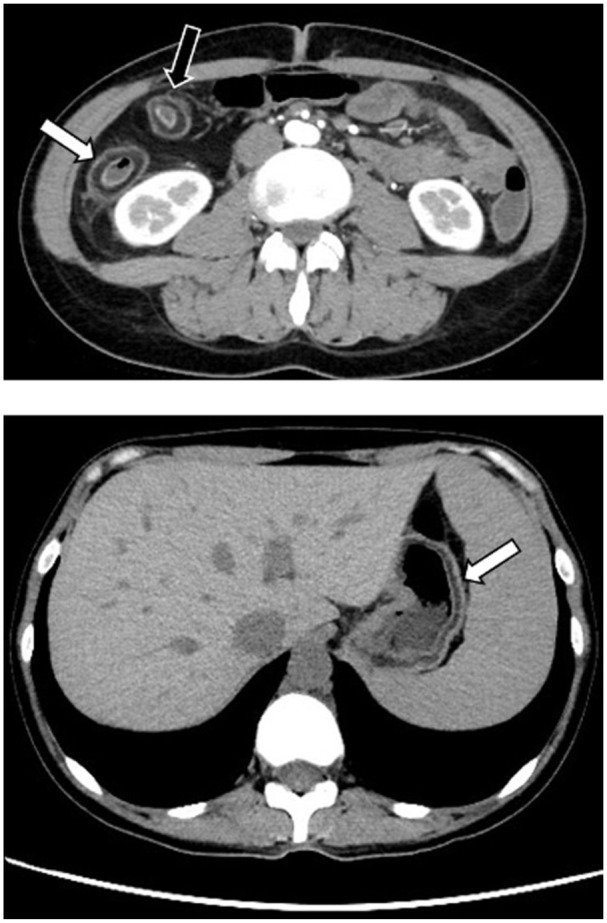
Axial CT section before treatment. The upper section of CT scan shows moderate bowel wall thickening with submucosal fat infiltration involving the ascending (white arrow) and proximal transverse colon (black arrow). In the lower section, there is also thickening of the gastric greater curvature.
